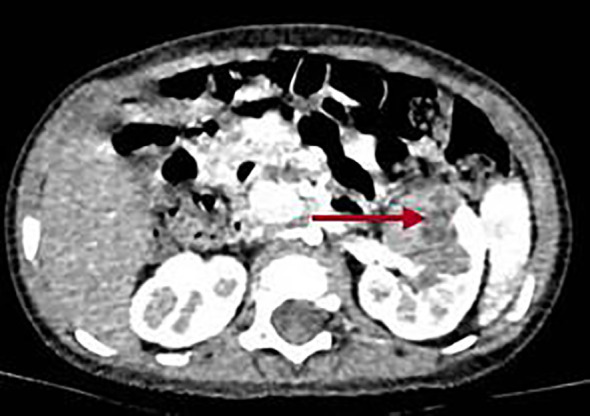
Image of the CT scan 5 months after the first surgery. The arrow points to the lesion in the left kidney.
